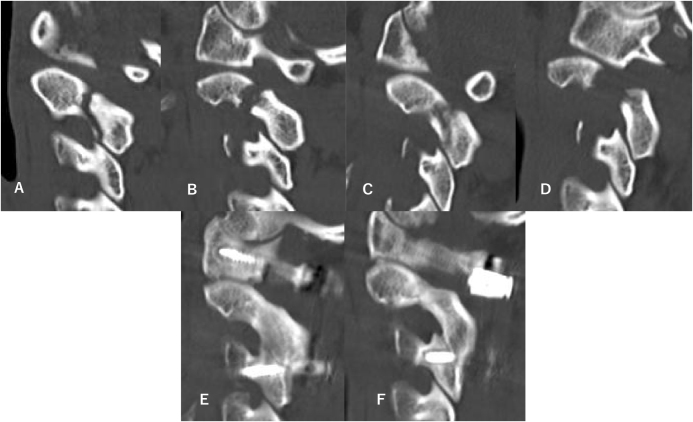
Preoperative and postoperative CT imaging of the C2 Hangman’s fracture. (A and B) Initial CT after injury shows bilateral pars interarticularis fractures with a gap of approximately 8 mm. (C and D) One-month follow-up CT demonstrates nonunion and persistent separation of the fracture site. (E and F) One-year postoperative CT confirms nearly complete bony fusion at the fracture site.
